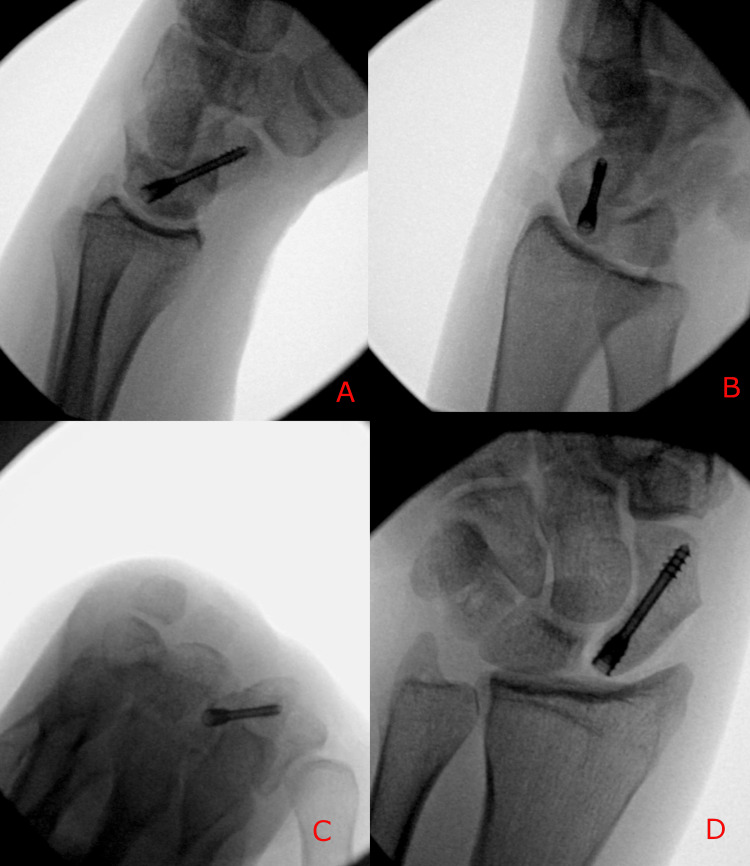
Intraoperative fluoroscopic images demonstrating scaphoid fracture fixation (A–D) Lateral, oblique, carpal tunnel views and posteroanterior radiographs showing placement of a headless compression screw across the scaphoid fracture.
